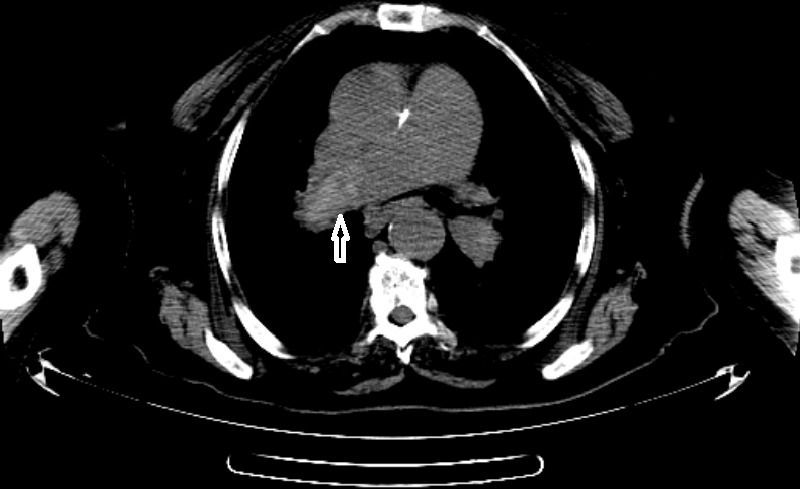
Unenhanced CT of the chest demonstrates hyperdense material within the right main pulmonary artery
